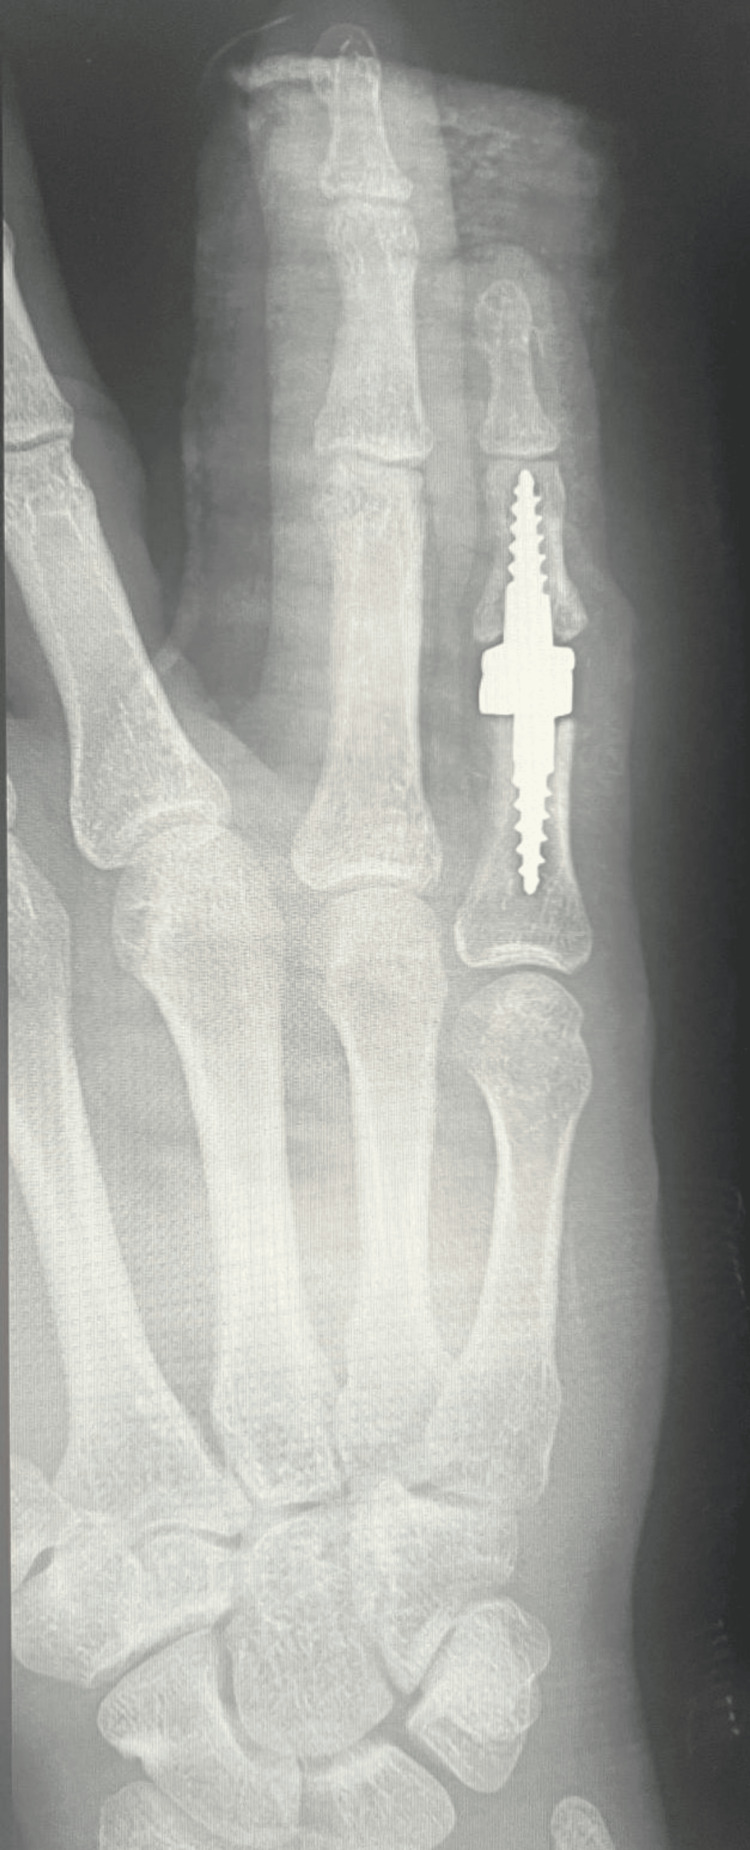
X-ray of the right hand in the AP projection on the first day after surgery AP: anteroposterior.
